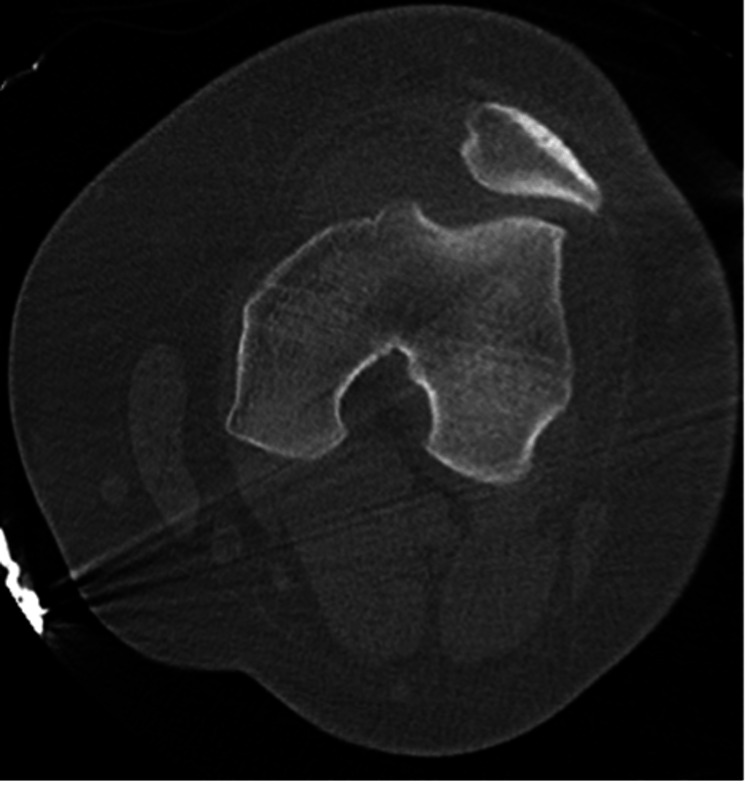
Post-reduction axial computed tomography image emphasizing the acute impaction defect of the left medial patella
